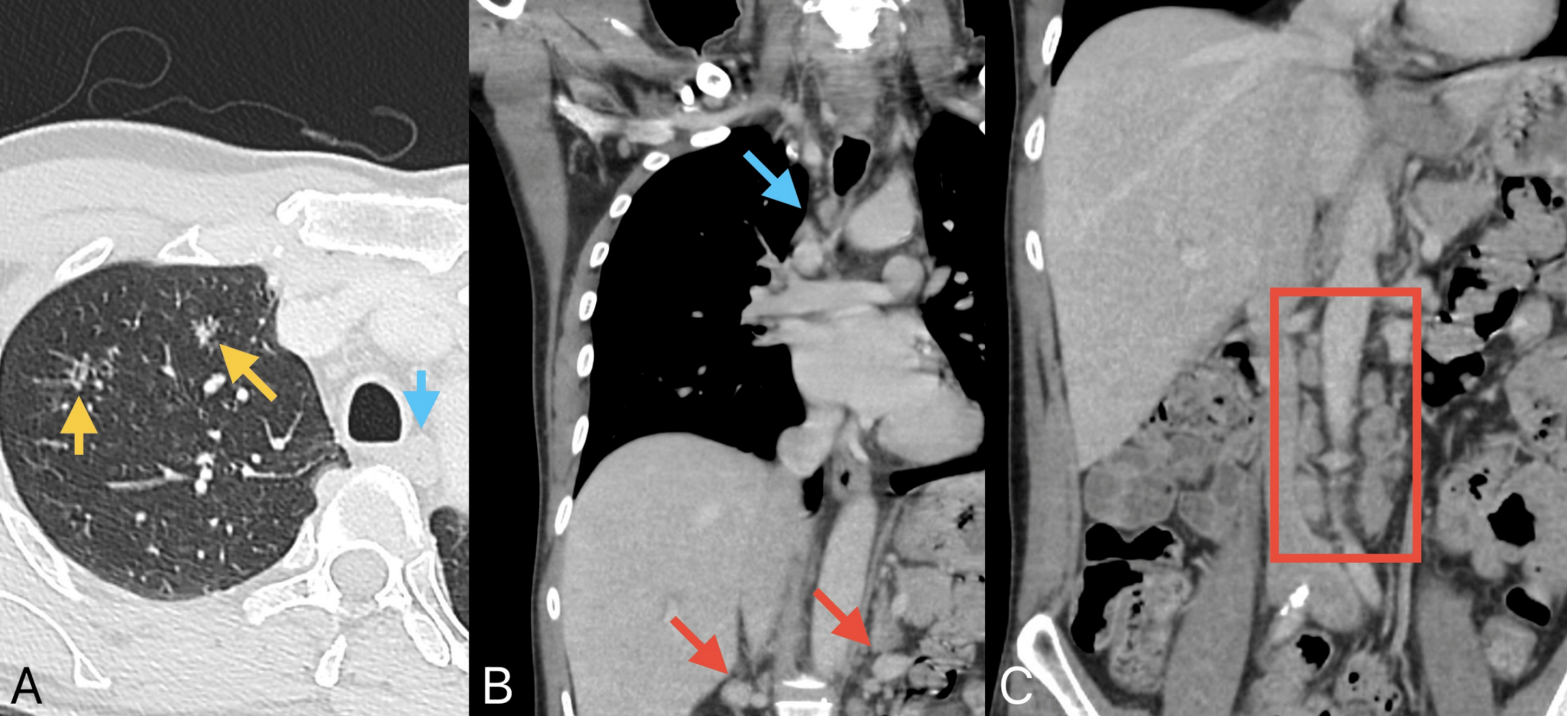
Contrast-enhanced CT scan of the chest (A and B) and abdomen (C) revealing ill-defined cavitary pulmonary nodules (yellow arrows), mediastinal lymph nodes (blue arrows), and lymph nodes in the porta hepatis (red arrows) and retroperitoneum (red box). CT: computed tomography
